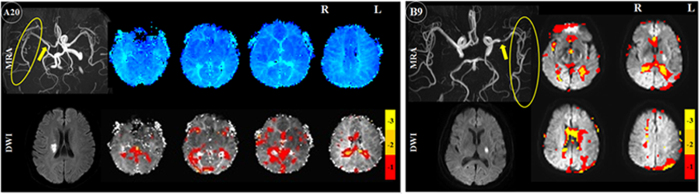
Patient A20 and B9, who had severe MRA stenosis/occlusion (yellow arrows) with abundant collaterals (yellow circles), no time delay regions were detected in the corresponding blood supply territory on TSA (A20, B9), nor TTP delay on DSC-PWI (A20). MRA = magnetic resonance angiography, DWI = diffusion weighted imaging. −1, −2, −3 in color bar indicate −1TR, −2TR, −3 TR time shift.
